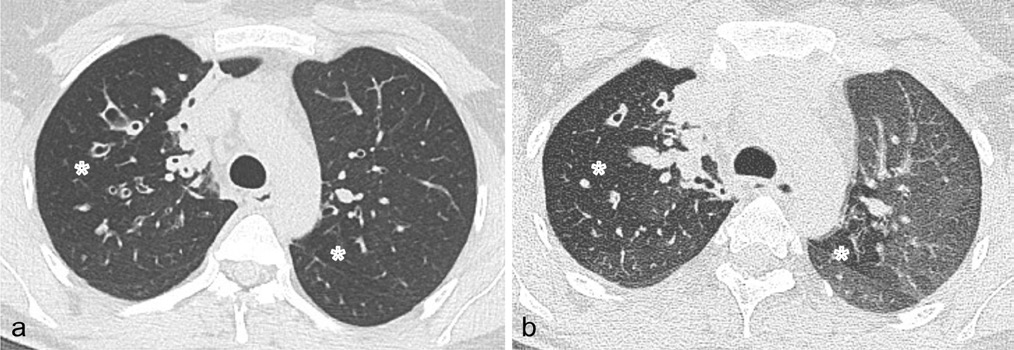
(a) Inspiratory axial CT image shows thick-walled bronchiectasis within areas of slightly decreased attenuation (asterisks) in the upper lobes. (b) Expiratory axial CT image acquired at the same level showed no changes in density of the hypo-attenuated areas (asterisks), demonstrating the presence of air trapping.
